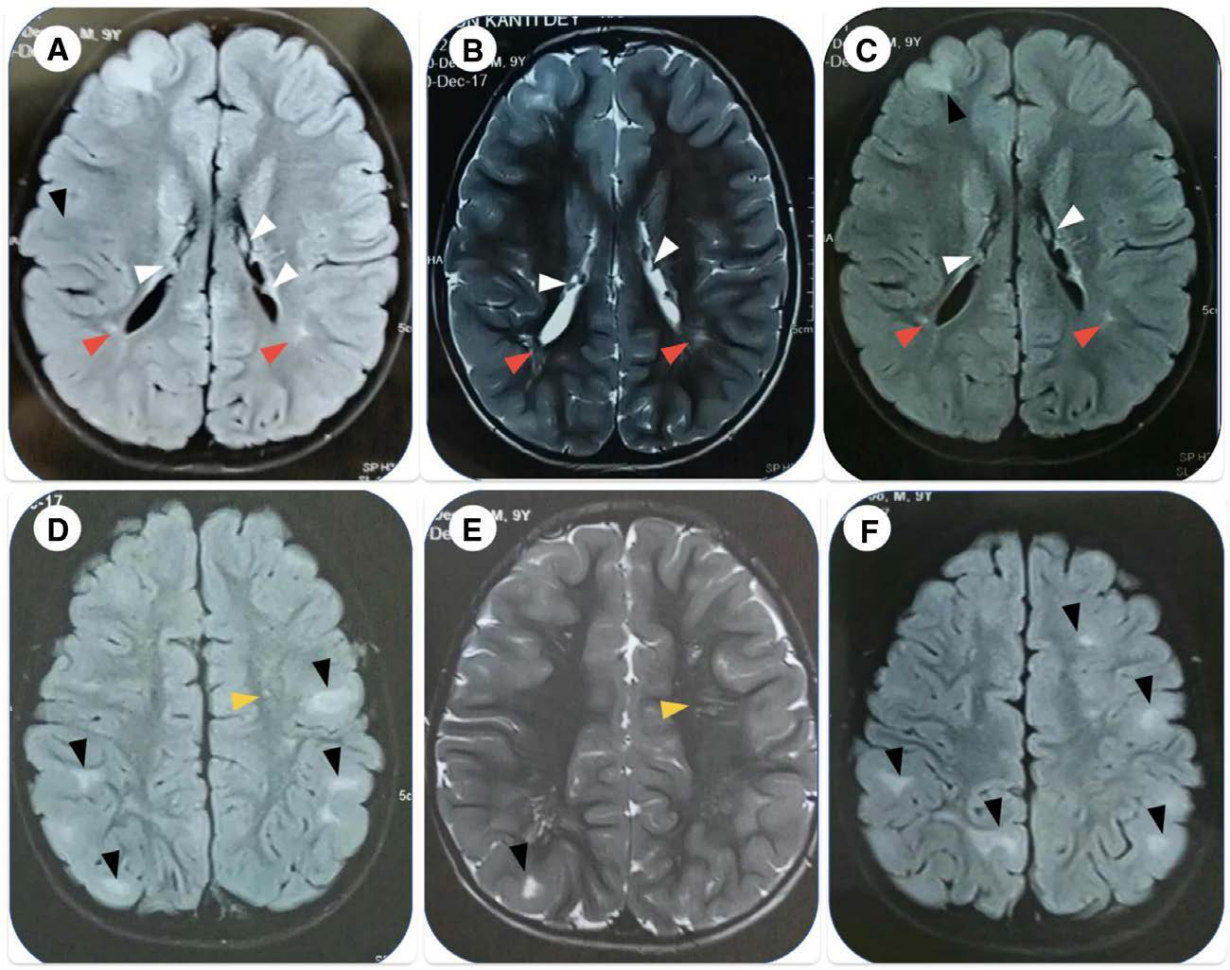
Axial MRI of the brain: cortical tubers (black arrowheads), subependymal nodules (white arrowheads), white matter lesions (red arrowheads), radial linear white matter lesions (yellow arrowheads). MRI = magnetic resonance imaging.
